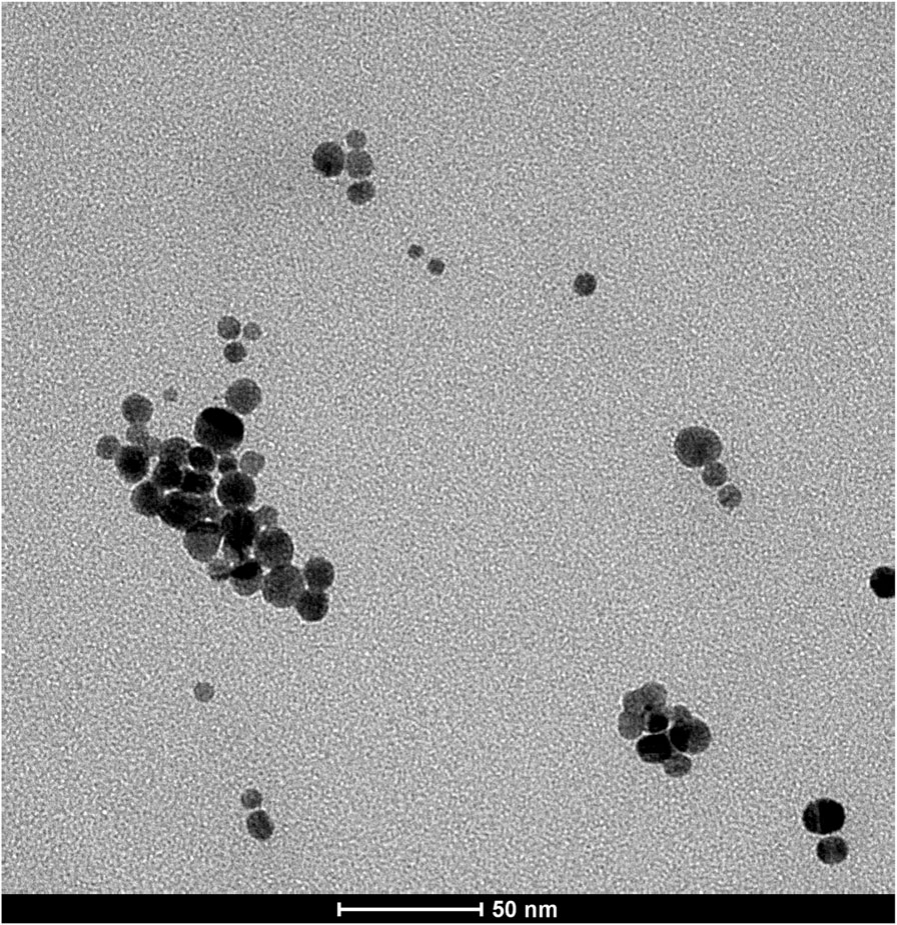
Representative TEM image of 10 nm CT-coated AgNPs. Particles were spherical in shape and mainly present as primary particles or loose agglomerates; no stable aggregates were visible (scale bar = 50 nm)
